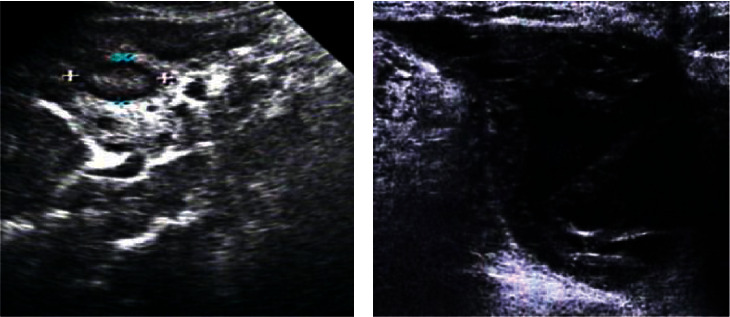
Images of ultrasound reexamination of some patients after surgery. (a) Reexamination result of a 56-year-old female patient. (b) Reexamination result of a 43-year-old female patient.
